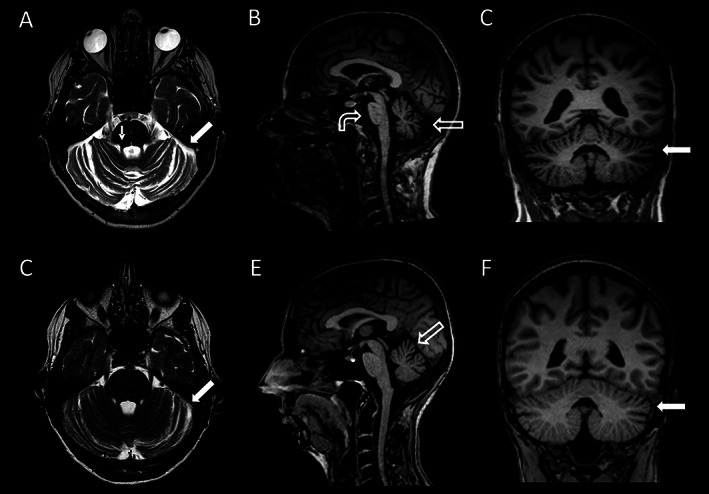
Fluid attenuated inversion recovery magnetic resonance imaging images in axial, coronal, and sagittal view. Mother (A–C), daughter (D–F). (A–C) showing advanced cerebellar atrophy involving the cerebellar vermis (open arrow in B), hemispheres (bold arrow in A and C), thinning of the upper cerebellar peduncle (thin arrow in A) and pontomesencephalic volume loss (curved arrow in B). (D–F) Showing accented slight left hemispheric thinning of the cerebellar folia (bold arrow in D and F) and predominantly upper vermal atrophy (open arrow in E).
